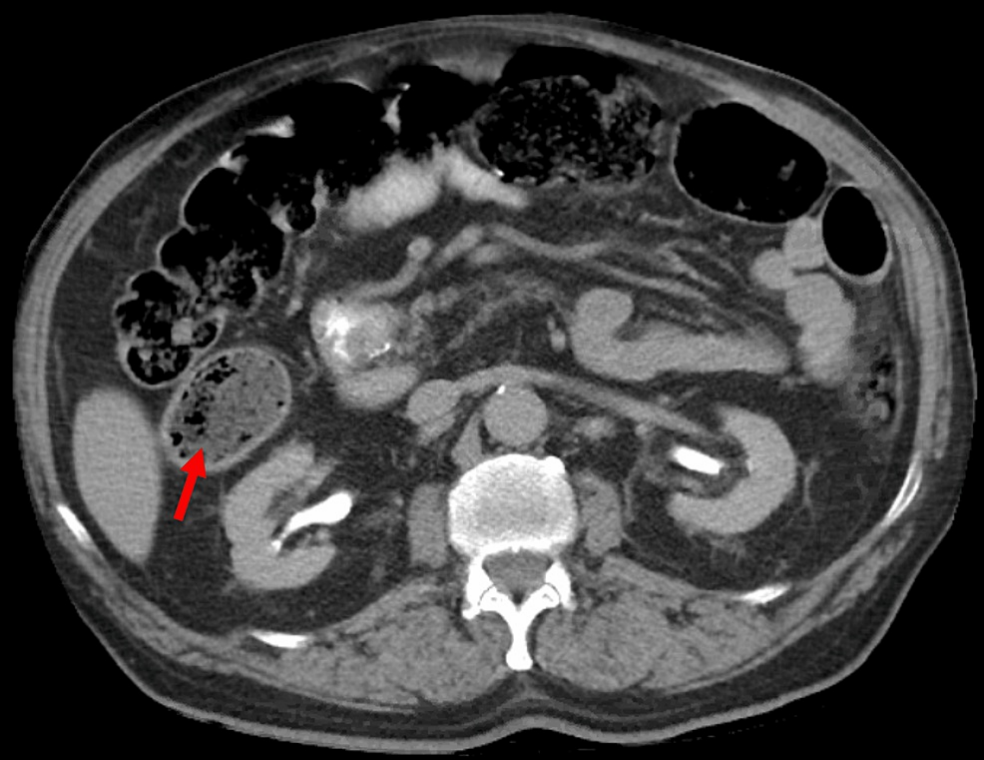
CT axial scan demonstrating cecal diverticulitis on the right (red arrow)
